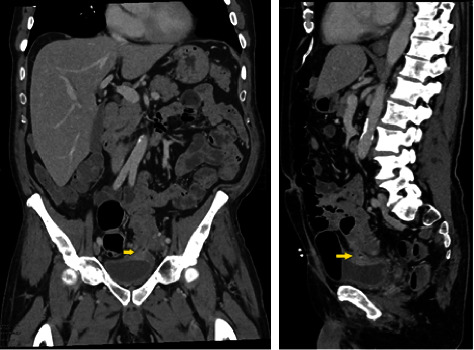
Sagittal and coronal CT imaging of colovesical fistula extending from the sigmoid colon to the left mid bladder.
